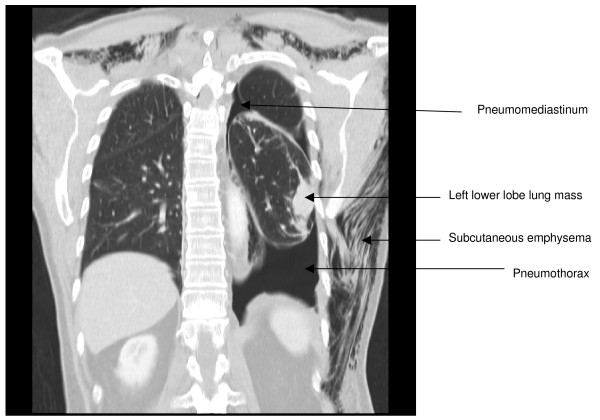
Coronal view of thoracic computed tomography scan showing pneumothorax, pneumomediastinum and subcutaneous emphysema which developed after iatrogenic pulmonary injury caused by Abrams pleural biopsy needle.
